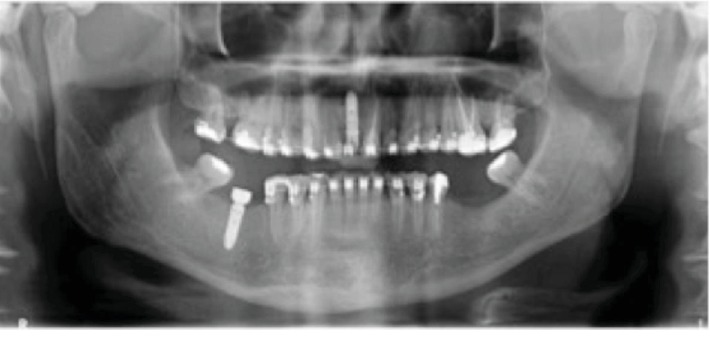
Postoperative panoramic radiograph without other complaints after 7 days.
